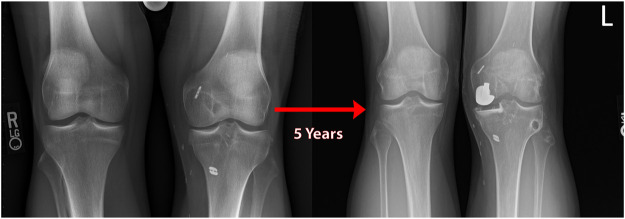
Standing bilateral radiographs of the knee of natural history patient #1 showing results of a unilateral knee arthroplasty used to treat medial compartment osteoarthritis over 5 years following an iatrogenic medial meniscal root tear (left knee).
